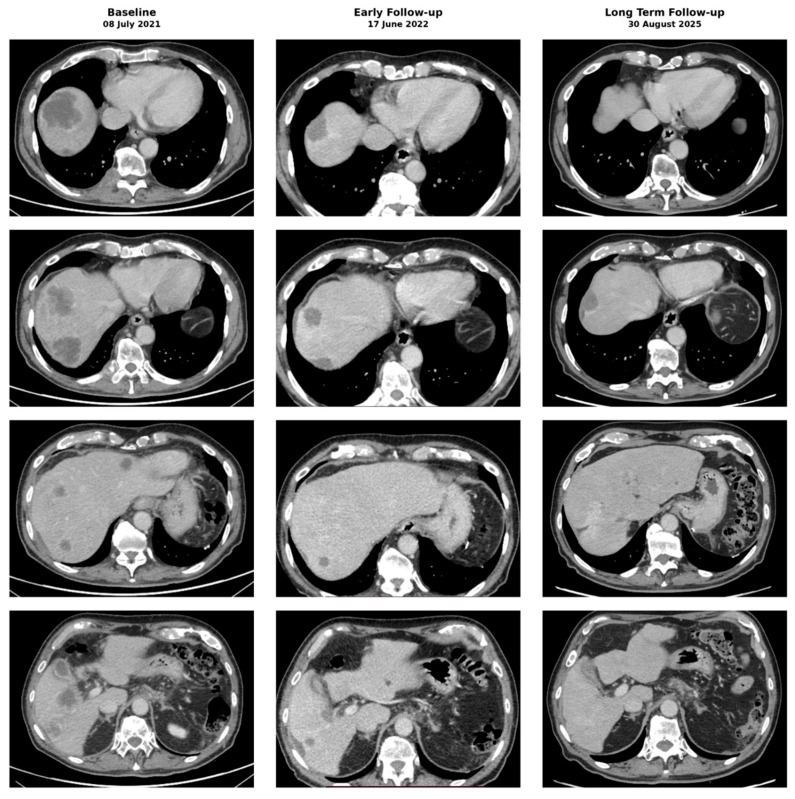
Radiological evolution of hepatic metastases during pembrolizumab treatment. Axial contrast-enhanced CT images obtained at baseline (8 July 2021), early follow-up (17 June 2022), and long-term follow-up (30 August 2025) are shown at corresponding anatomical levels. Baseline imaging shows multiple hepatic metastases. Early follow-up (17 June 2022) imaging demonstrates a partial response, with persistent disappearance of the target lesions at long-term follow-up, consistent with a sustained complete radiological response.
